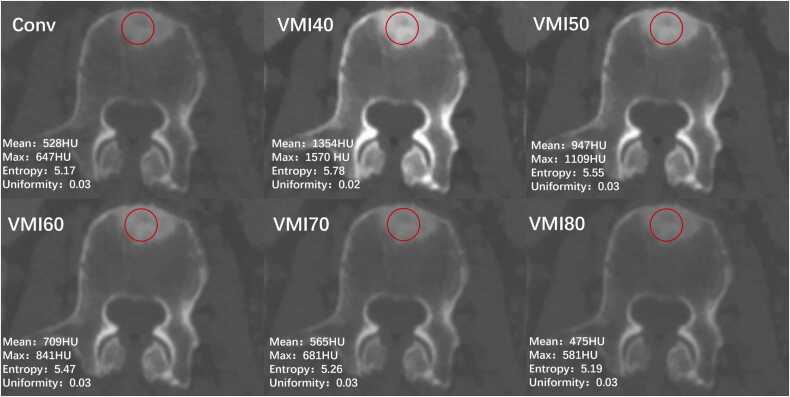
Axial CT images in a 71-year-old male patient with OBM from gastric cancer. Maximum, minimum, entropy, and uniformity of lesions measured on conventional and VMI40–80 images. CT, Computed tomography; OBM, osteoblastic bone metastasis.
